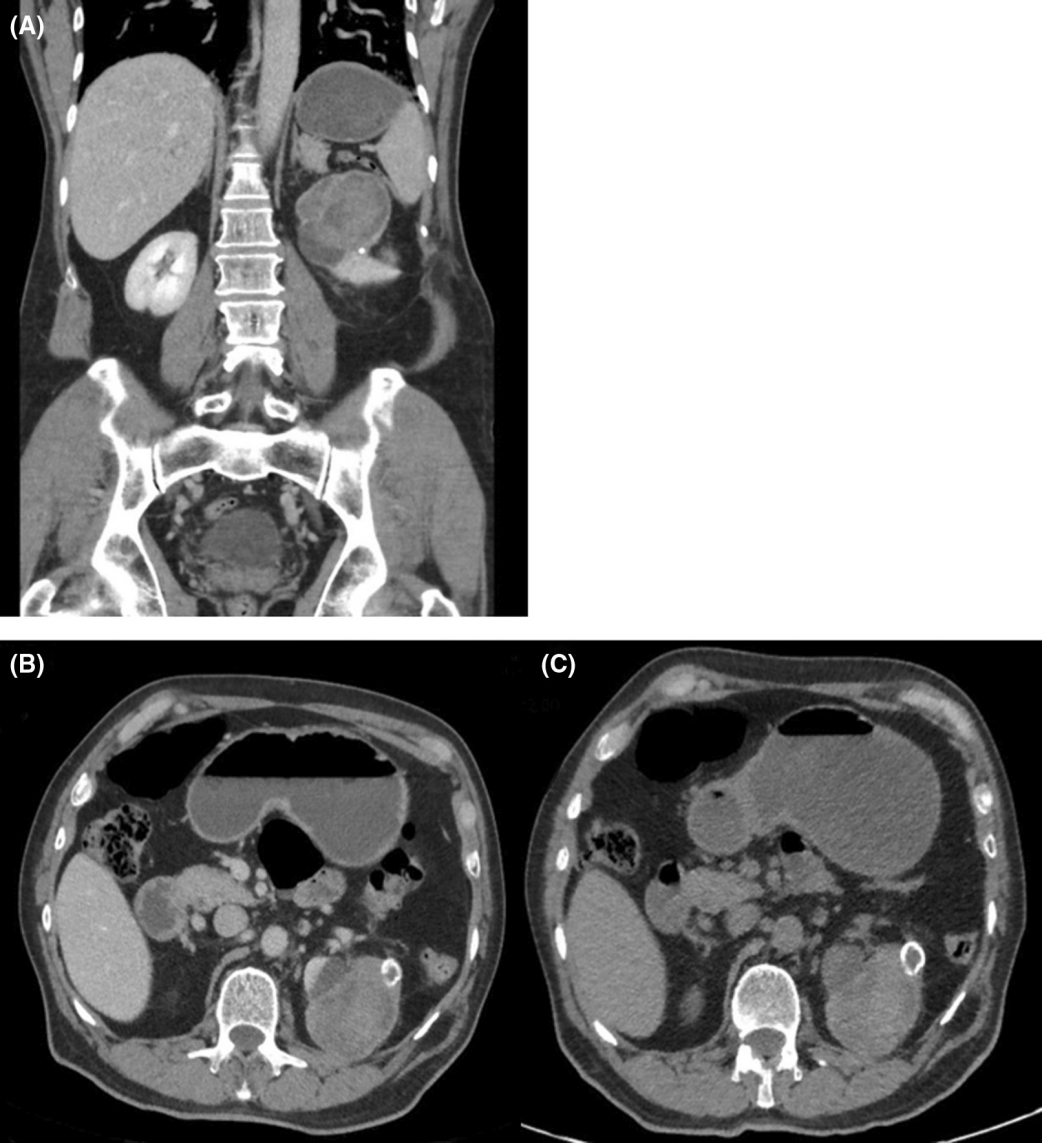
(A) Coronal contrast‐enhanced CT image demonstrates a large, lobulated cystic mass in the upper pole of the left kidney. (B, C) Axial contrast‐enhanced CT (B) and non‐contrast CT (C) image shows a well‐circumscribed cystic lesion in the upper pole of the left kidney.
